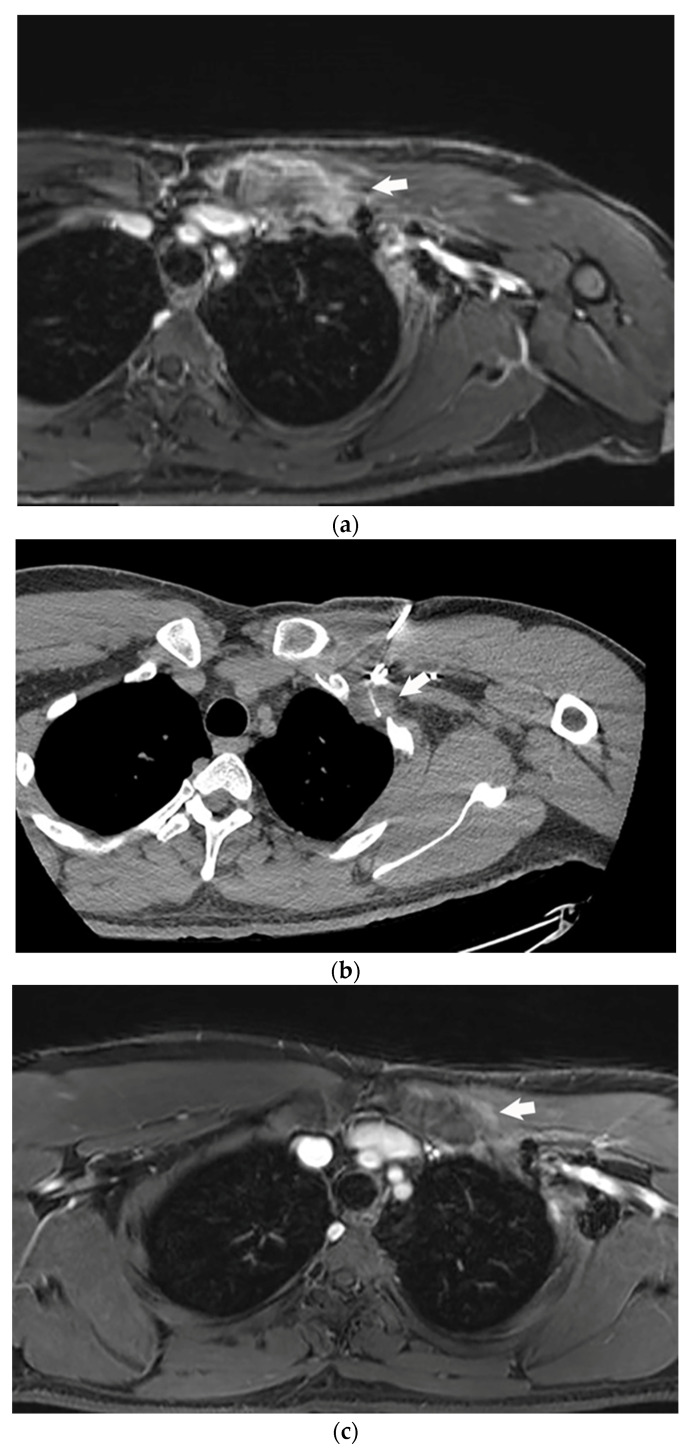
Desmoid tumor patient following surgical resection and cryoablation of residual desmoid tumor. (a) Selected post contrast magnetic resonance imaging image demonstrating avid enhancement of residual desmoid tumor (arrow) following surgical resection. The patient also continued to have left upper extremity pain, which was significantly limiting his quality of life. (b) Intra procedural computed tomography during percutaneous cryoablation of residual desmoid tumor (arrow) following prior resection. Hypodense ice ball, which roughly approximates the anticipated ablation zone, is seen surrounding cryoablation probe (arrow), within the desmoid tumor. (c) Post contrast magnetic resonance imaging demonstrating no evidence of residual desmoid tumor following cryoablation (arrow).
